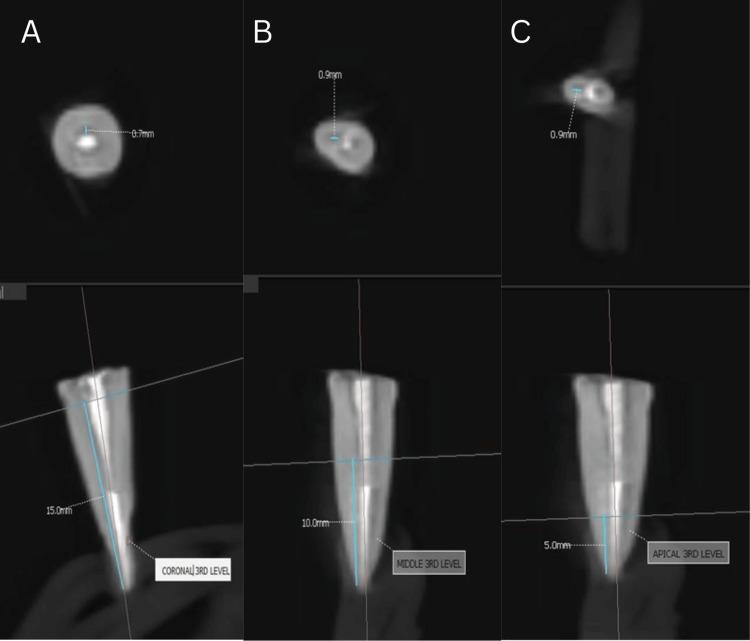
CBCT analysis of single cone technique. CBCT images showing obturation with the single-cone technique in the (A) coronal third, (B) middle third, and (C) apical third.
